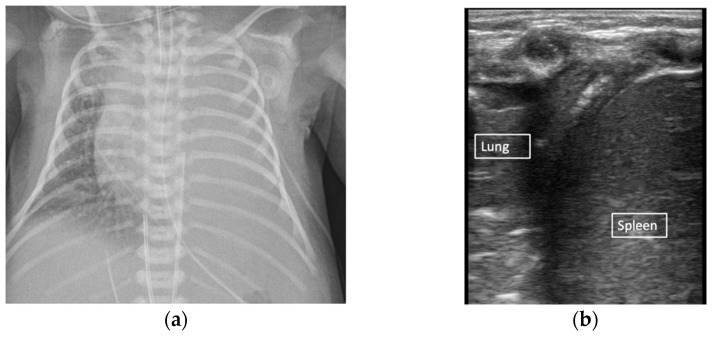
Effusion vs complete atelectasis. Large effusion suspected on chest X-ray (a). B-mode demonstrating complete atelectasis of the left lung without large pleural effusion (b).
